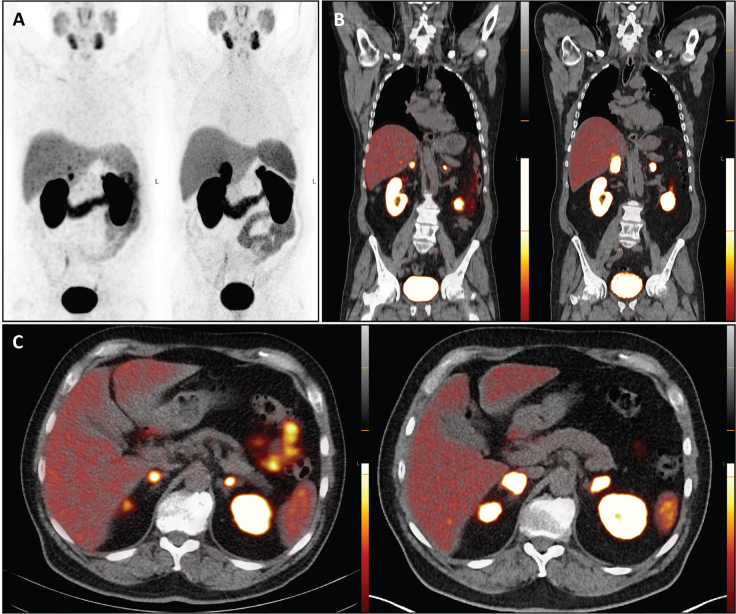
The PET/CT images with ^68^Ga-PSMA depict maximum intensity projection (A), coronal (B), and transversal (C) views. The images on the left are from February 2022, while those on the right are from March 2023. A visual comparison reveals disease progression in both adrenal glands over the 13-month period, despite ongoing androgen deprivation therapy. Specifically, in March 2023, there is increased PSMA uptake in the adrenal masses compared to February 2022. For instance, while the radiotracer uptake in the right adrenal gland appears bifocal and small in the February 2022 scan, a single intense and more extensive uptake is evident in the March 2023 scan. Notably, no new metastases or local recurrence were detected after this timeframe.
